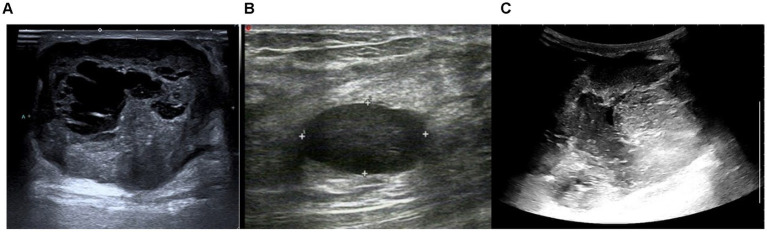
(A) Squamous cell carcinoma: Ultrasound image of an 80-year-old woman reveals a large, predominantly solid mass measuring 5.5 × 4.2 cm. The tumor displays an irregular shape with indistinct, angular margins. The focal anechoic area is suggestive of cystic degeneration or necrosis. (B) Spindle Cell Carcinoma: Ultrasound image of a 56-year-old woman presenting with a 2.1 × 1.2 cm mass. The lesion exhibits benign-appearing features, including a regular oval shape, circumscribed margins, and a parallel orientation. The internal echo texture is homogeneous and hypoechoic, closely mimicking a fibroadenoma. This pseudo-benign appearance can lead to initial diagnostic underestimation, as shown by some spindle cell lesions being classified as BI-RADS 3 or 4A. (C) Mixed type: Ultrasound image of a 42-year-old woman with a giant 11.2 cm mass. The tumor presents as a lobulated mass with microlobulated margins, reflecting its expansive growth pattern. It appears as a predominantly solid, heterogeneous mass with a complex internal architecture and scattered punctate or patchy echogenic foci.
